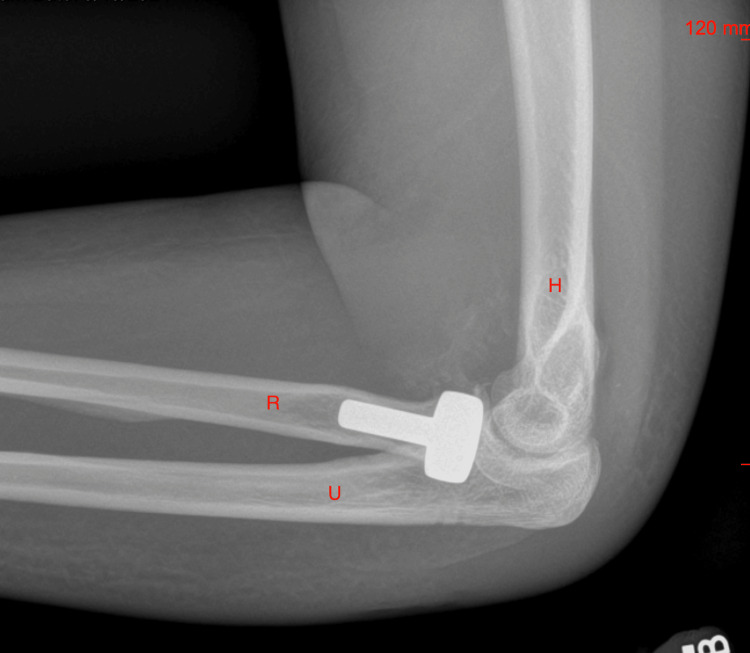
An X-ray of the left elbow on postoperative day 15. H: humerus; R: radius; U: ulna
